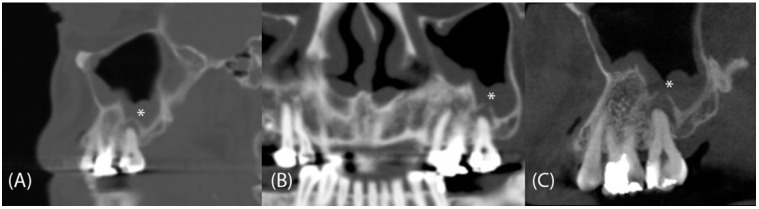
CPR and dental CBCT could identify more dental pathologies than conventional CT images. (A) The parasagittal CT view identified a periapical lesion of a left maxillary molar (no. 27, asterisk); however, both (B) CPR and (C) CBCT revealed a co-existing fistula (*).
